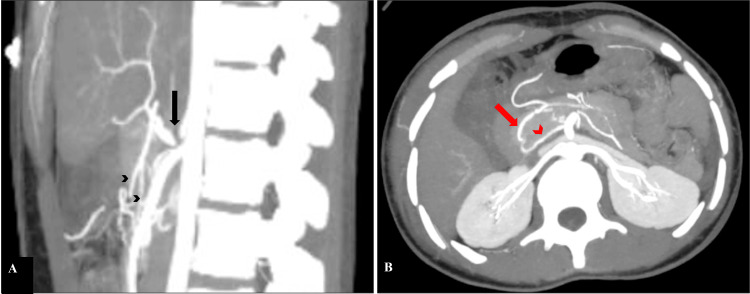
Celiac artery stenosis and the collateral pancreaticoduodenal arcades Contrast-enhanced CT scan of the abdomen, sagittal (A) and axial (B) sections in maximum intensity projection (MIP), shows focal interruption (black arrow) of the contrast column near the Ostia of the celiac trunk with normal opacification of the distal celiac trunk and its branches (black arrowheads). Image B depicts the course of pancreaticoduodenal arcades. The anterior arcade (red arrow) is anteriorly and laterally placed, and runs in the pancreaticoduodenal groove. The posterior arcade (red arrowhead) is posteriorly and medially located.
